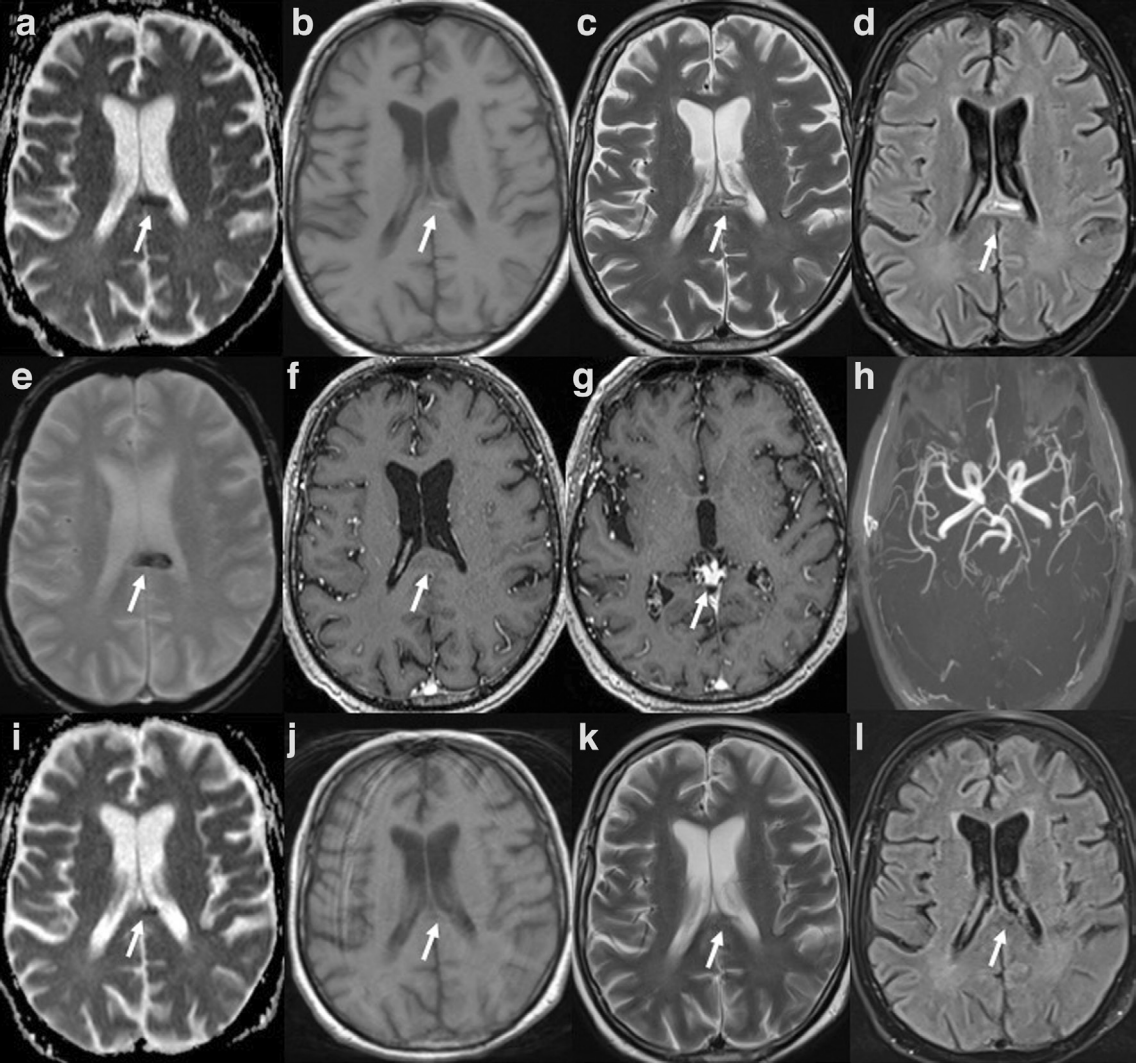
MRI findings in a 73-year-old male with COVID-19 (top two rows): (A) ADC) map shows a midline, hyporintense lesion in the splenium of the corpus callosum (CLOCC); (B) *T*_1_ weighted image showing slight increase in the signal; C) *T*_2_ weighted image show central hyperintensity with surrounding iso/hypointense rim; (D) in FLAIR, the lesion appears hyperintense, more hyperintense centrally; (E) the lesion in gradient-echo image appears hypointense; (F) no true gadolinium enhancement is noticed; (G) T1-MPRAGE images did not show any filling defect in the deep cerebral veins and (H) 3D-TOF was negative for cerebral aneurysms. MRI findings at follow-up (third row): (I) ADC map shows progressive residual area of restriction; (J) *T1*W image shows a small hypointense area; (K) *T2*W and (L) FLAIR show quasiresolution of the initial lesion likely evolving into a small malacic area. 3D-TOF, three-dimensional time of flight; ADC, apparent diffusion coefficient; FLAIR, fluid attenuated inversion recovery.
